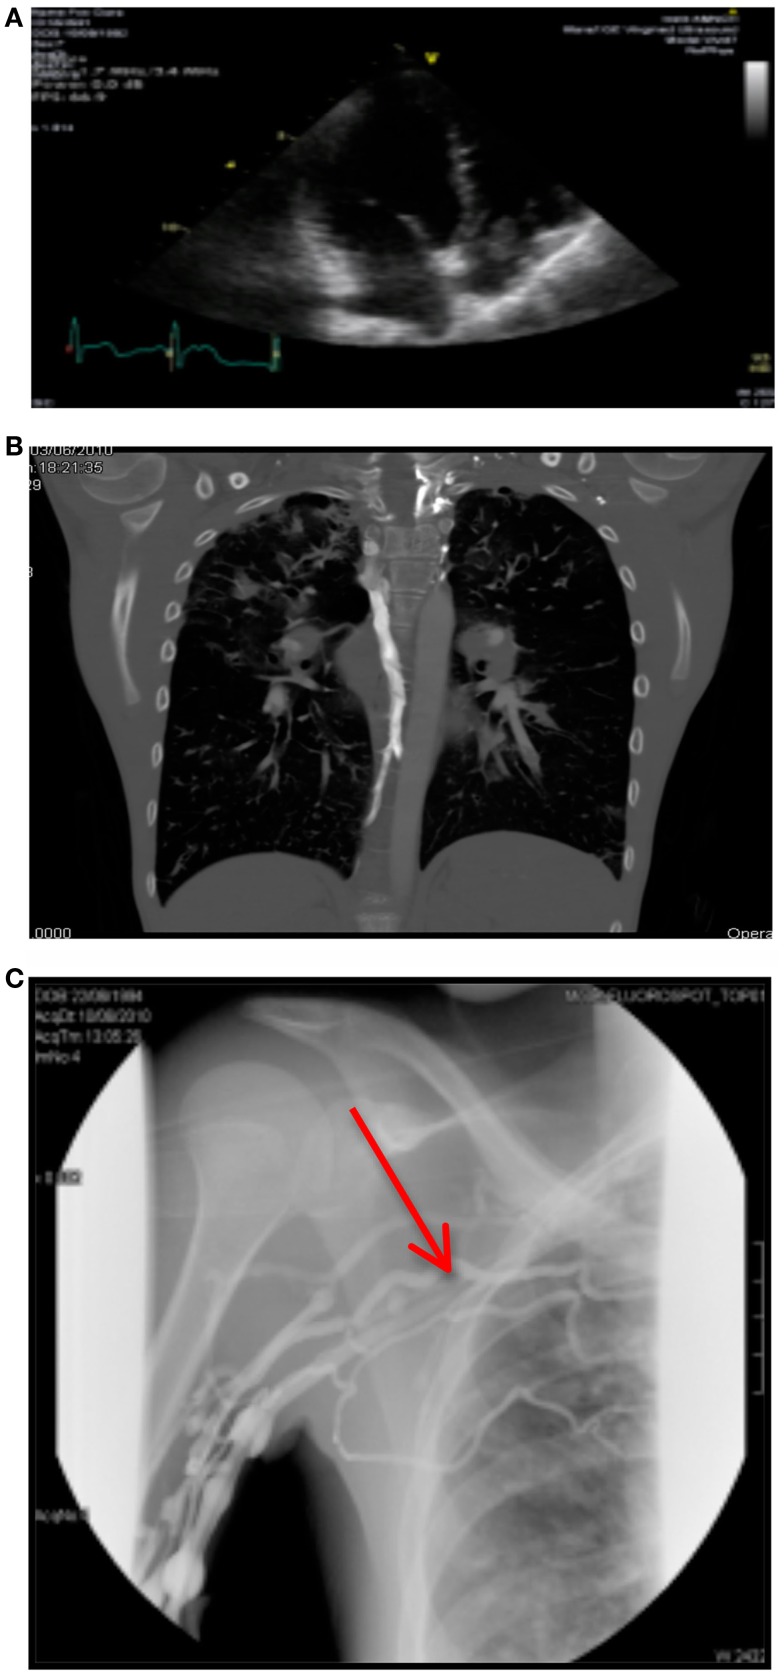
(A) Right atrial thrombus on echocardiography. (B) CTPA showing bilateral arterial filing defects. (C) Venogram showing complete obstruction of right subclavian vein.
